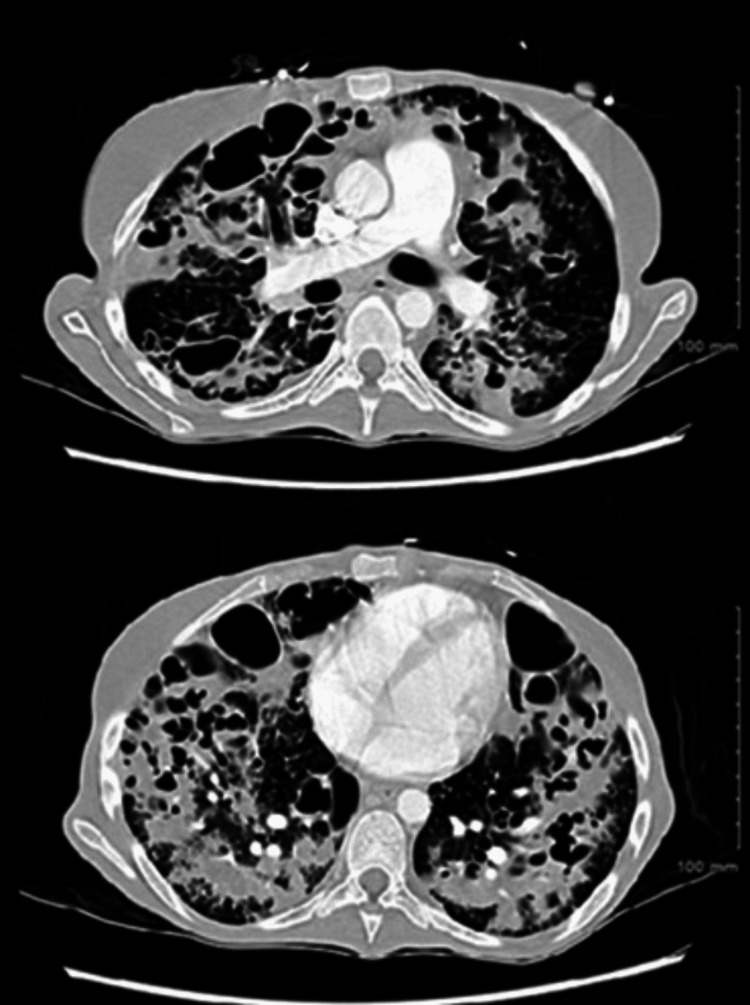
Varicose bronchiectasis CT scans of the chest with contrast showed extensive severe cystic and varicose bronchiectasis throughout the lung.
